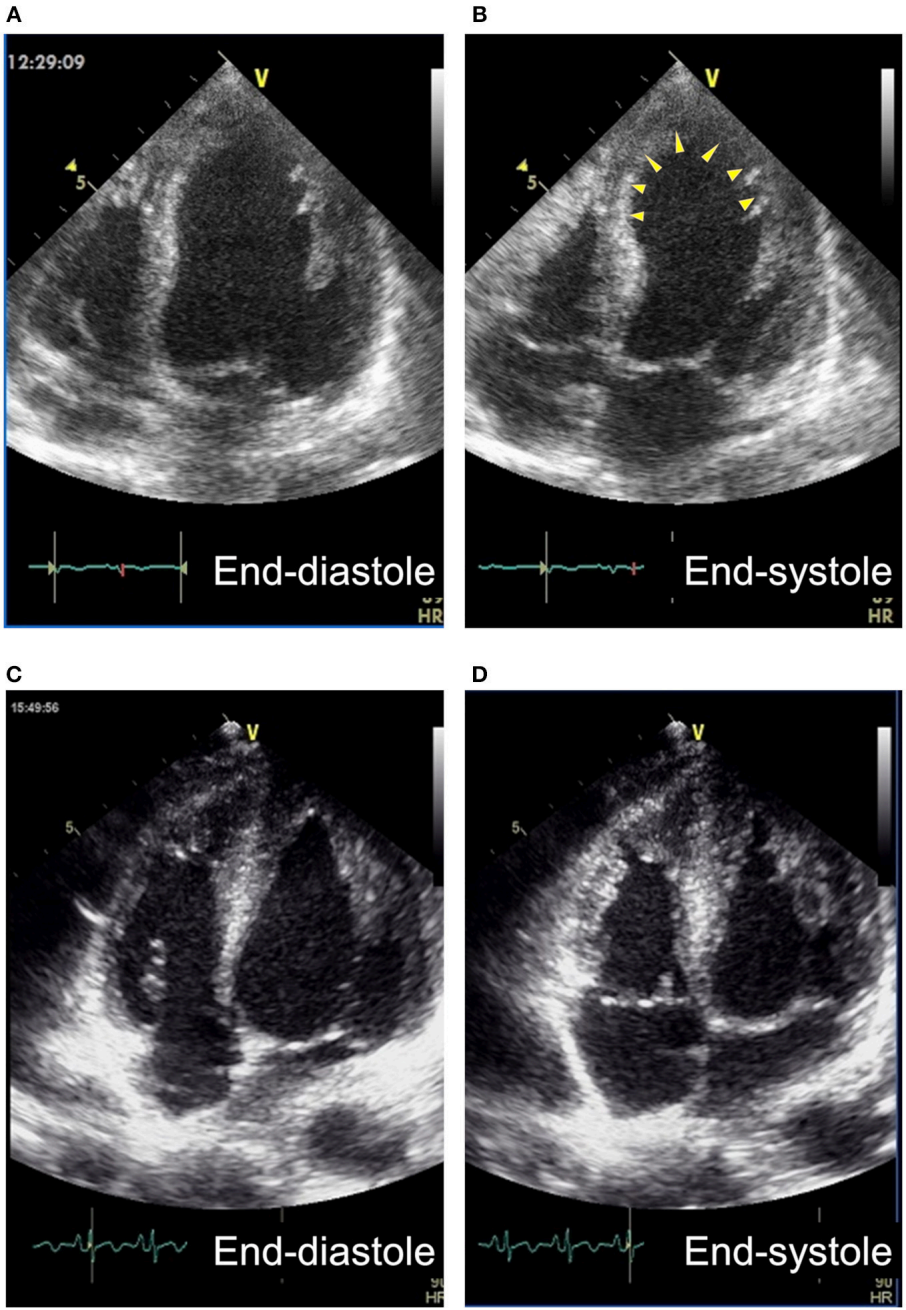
Apical 4-chamber views of transthoracic echocardiography of Case 3. (A,B) The broad anterior wall including apex (arrowheads) showed akinesis at the onset. (C,D) Myocardium of the region became hypertrophied and showed normal wall motion 2 weeks after the onset.
